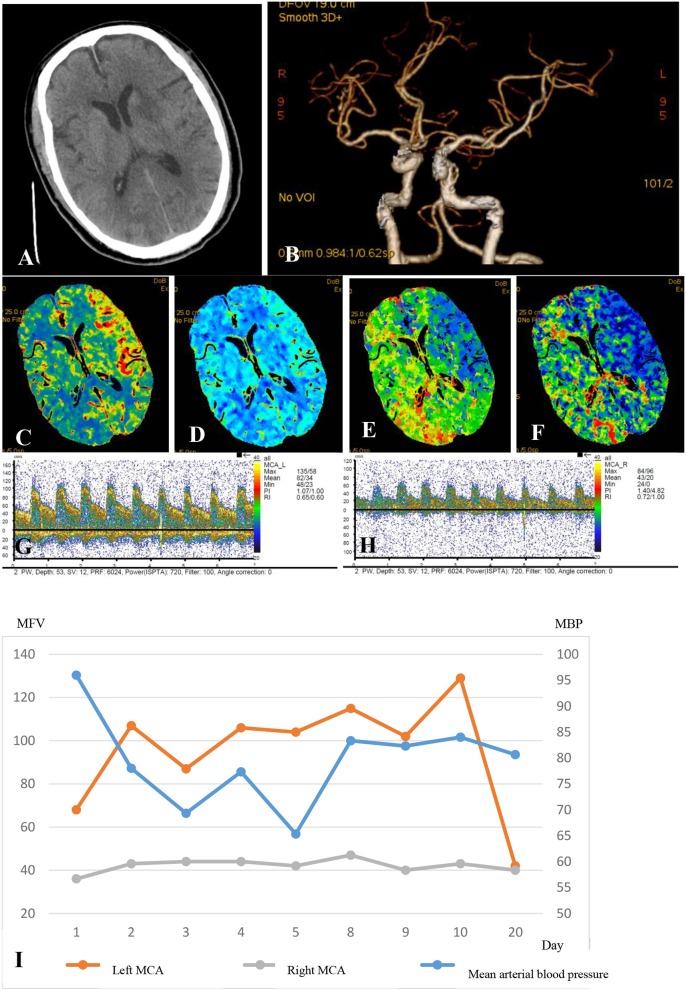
A 75-year-old man who received a successful mechanical thrombectomy in the C1 segment of the left ICA 3 h 50min after the onset of AIS. The NIHSS score at baseline was 22. Twenty-four hours after the recanalization, the NIHSS score slightly decreased to 20, and a multimodal hemodynamic evaluation was performed. The non-contrast CT scan shows the hypointense infarct core in the left perforating MCA territory (A). The left ICA and MCA reappeared entirely on CTA, and the M1 segment of the left MCA showed no stenosis (B). The CTP series showed markedly increased CBF (C), mildly increased CBV (D), significantly decreased MTT (E), and higher TTP (F) in the left MCA territory compared to those in the infarct core. The mean flow velocity of the left MCA increased by 100% compared to that on the right side at the same depth (G, left MCA; H, right MCA). The patient was considered as having the hyperperfusion syndrome. The systolic blood pressure was controlled at <110 mmHg until the flow velocity of the left MCA was restored to the normal in 20 days (I). The patient had no hemorrhagic transformation or epileptic seizures but had an mRS score of 5 at 90 days, although the final infarct volume was only 12ml. Electroencephalographic monitoring was also performed (data not shown).
